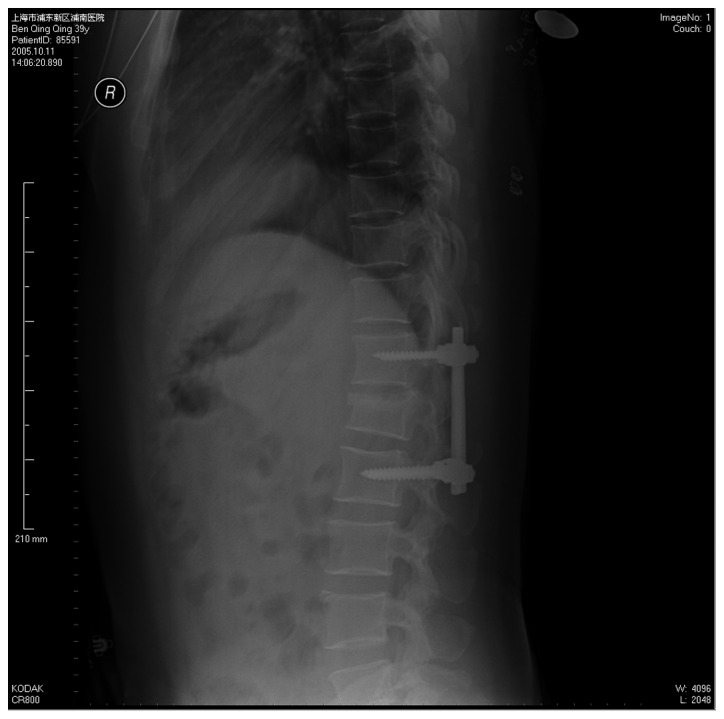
Lateral radiograph following dual-plane fixation of an L1 fracture for one year.
